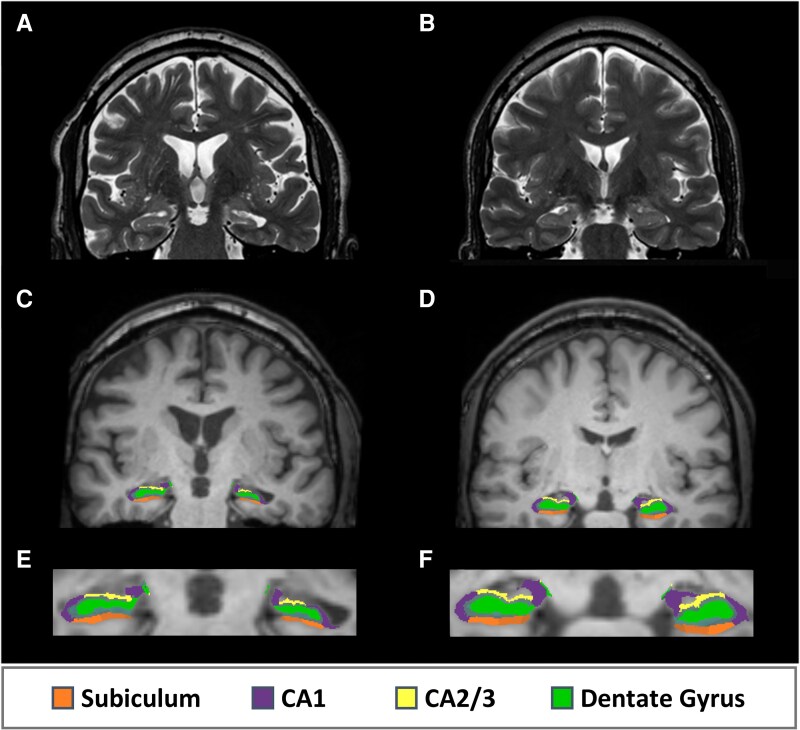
MRI image of hippocampal atrophy of the patients. Cranial MRI images of one representative LGI1–LE patient (left row: A, C, E) and a matched control participant (right row: B, D, F). A and B: T2; C and D: T1 with marked HC regions for volumetric analysis. E and F: T1 magnification. The volumetry of a LGI1 patient shows bilateral hippocampal atrophy. Note: the hippocampal sclerosis is accompanied by an internal structural loss primarily of the layers for CA3 and DG.
